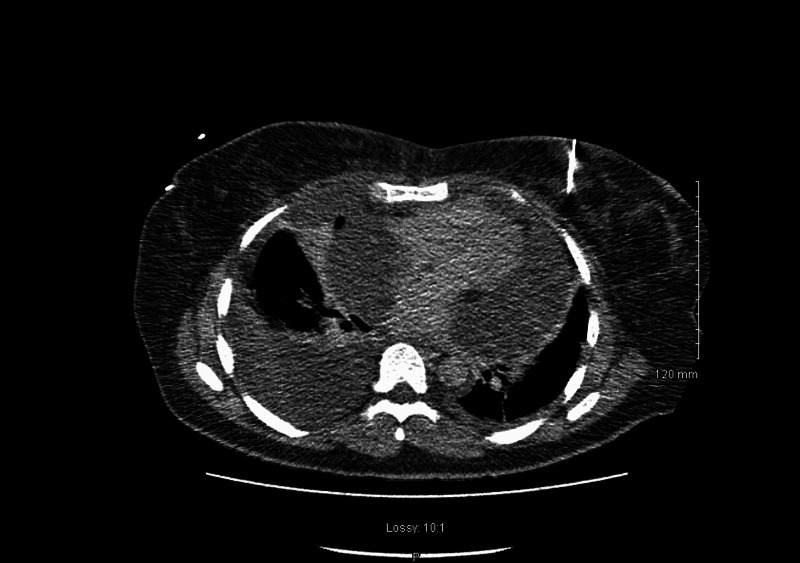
CT thorax showing large volume pericardial effusion with tamponade and bilateral plural effusion more prominent on right lung.
